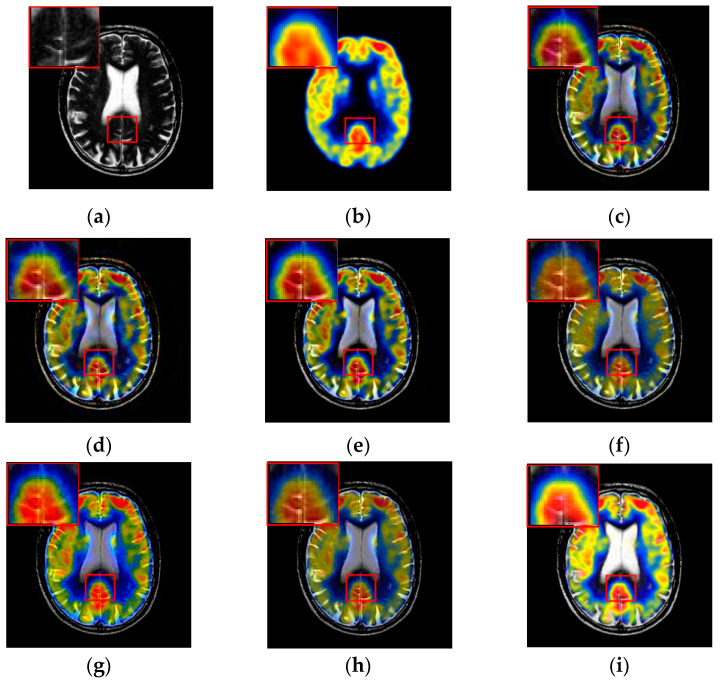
Fused results of MRI/PET medical images by different methods. (a) MRI; (b) PET; (c) DCTWT; (d) Curvelet; (e) NSCT; (f) Liu-ASR; (g) Kim; (h) Zhu; (i) Proposed.
